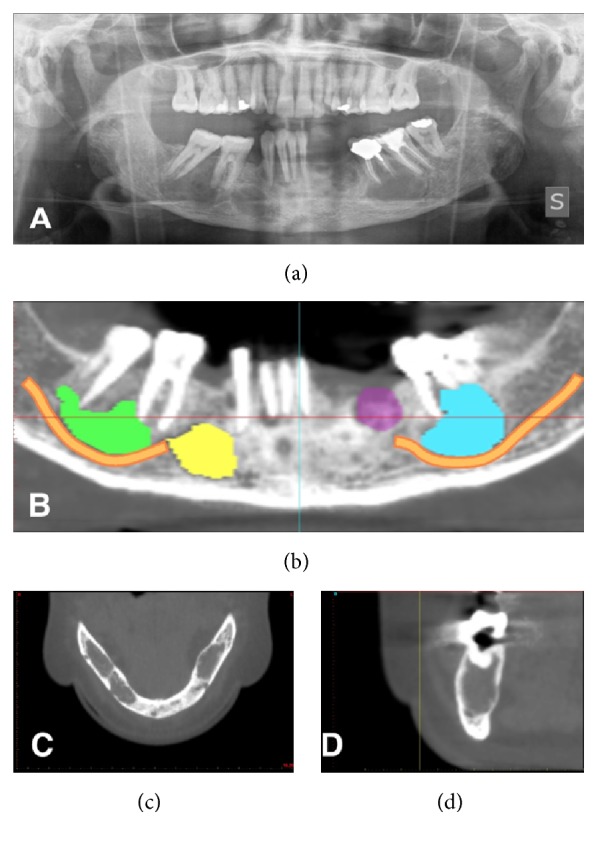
Radiographic appearance after six months. (a) Multiple osteolytic areas involving the mandible bilaterally revealed by another orthopantomogram (b–d) and CT scan six months after.
